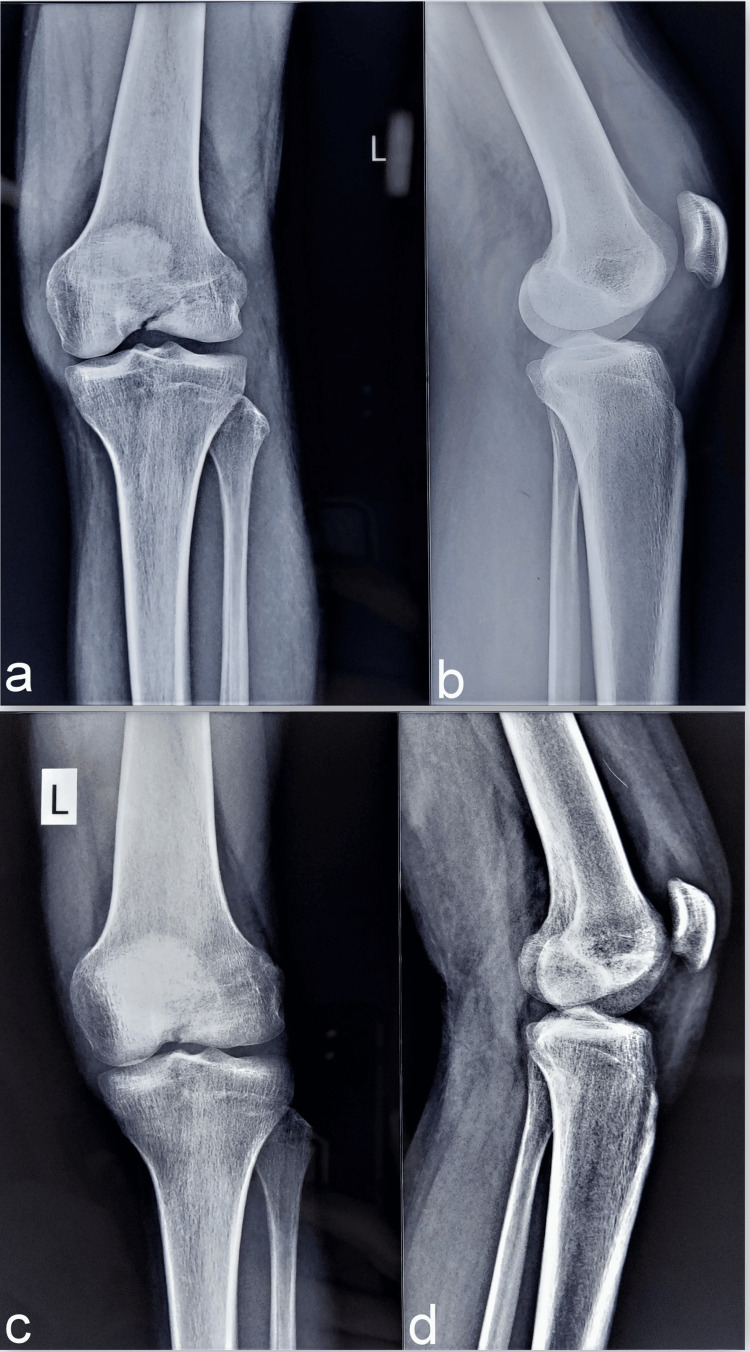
The knee orthogonal radiographs (a, b) show the undisplaced and incomplete fracture in the intercondylar region of the knee. The fracture is not traversing the distal femoral condyles. The radiographs at the final follow-up (c, d) show the healing of the fracture following conservative management.
